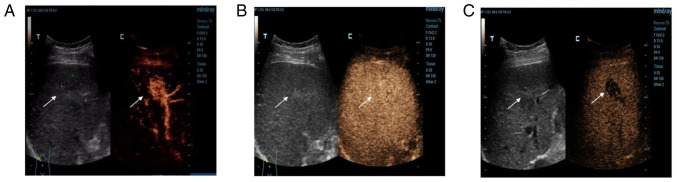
Contrast-enhanced ultrasound examination of liver segment 5 lesion. (A) The development time of the hyperechoic mass (arrow) was significantly earlier than that of the hepatic parenchyma during the arterial phase, and the range of the mass was wider than that of conventional images. (B) The mass (arrow) was continuously observed and developed in the portal vein phase. (C) The mass (arrow) in the delay period was not fully clear until 6 min later.
